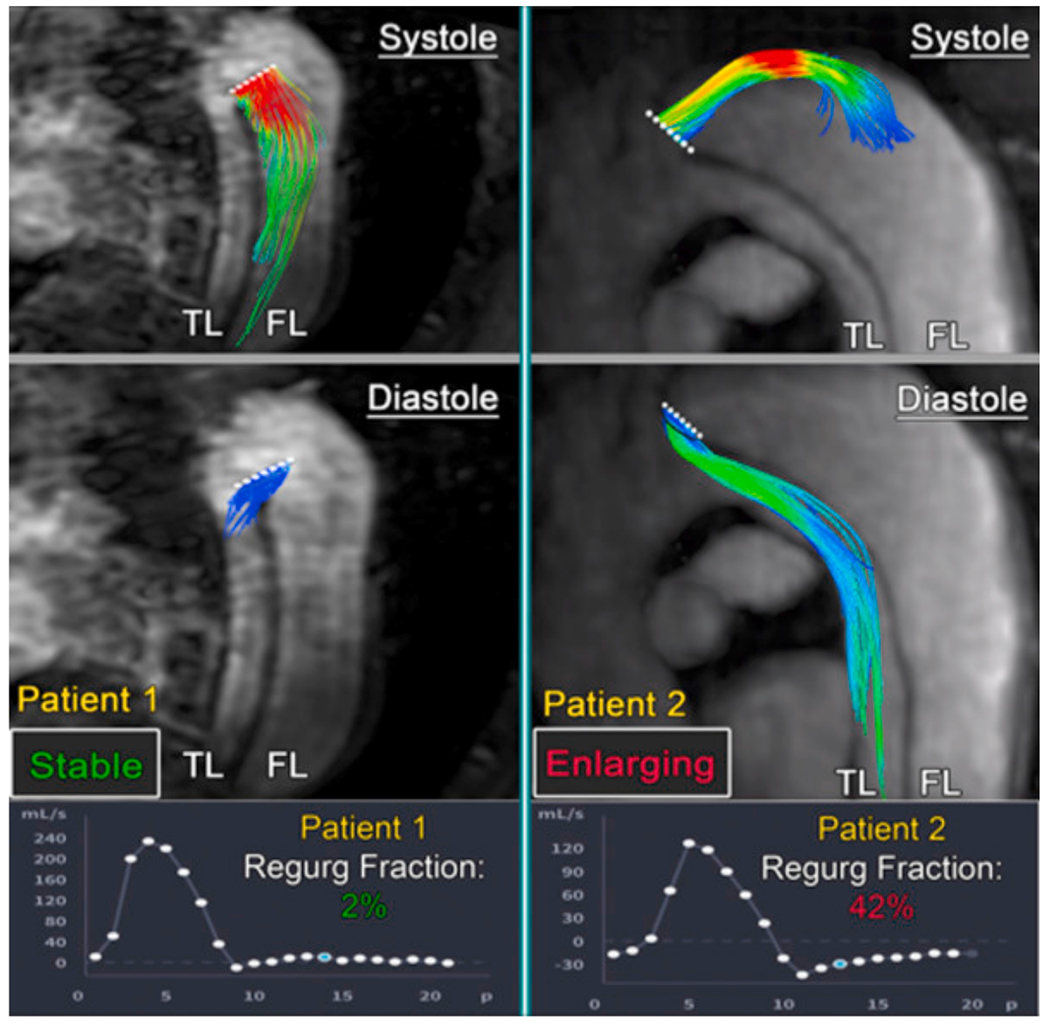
3D PC-MRI results of two representative patients with a history of a stable and dilating false lumen (‘FL’) (‘TL’: true lumen). Figure is reprinted from Burris et al. [514] with permission from Elsevier.
